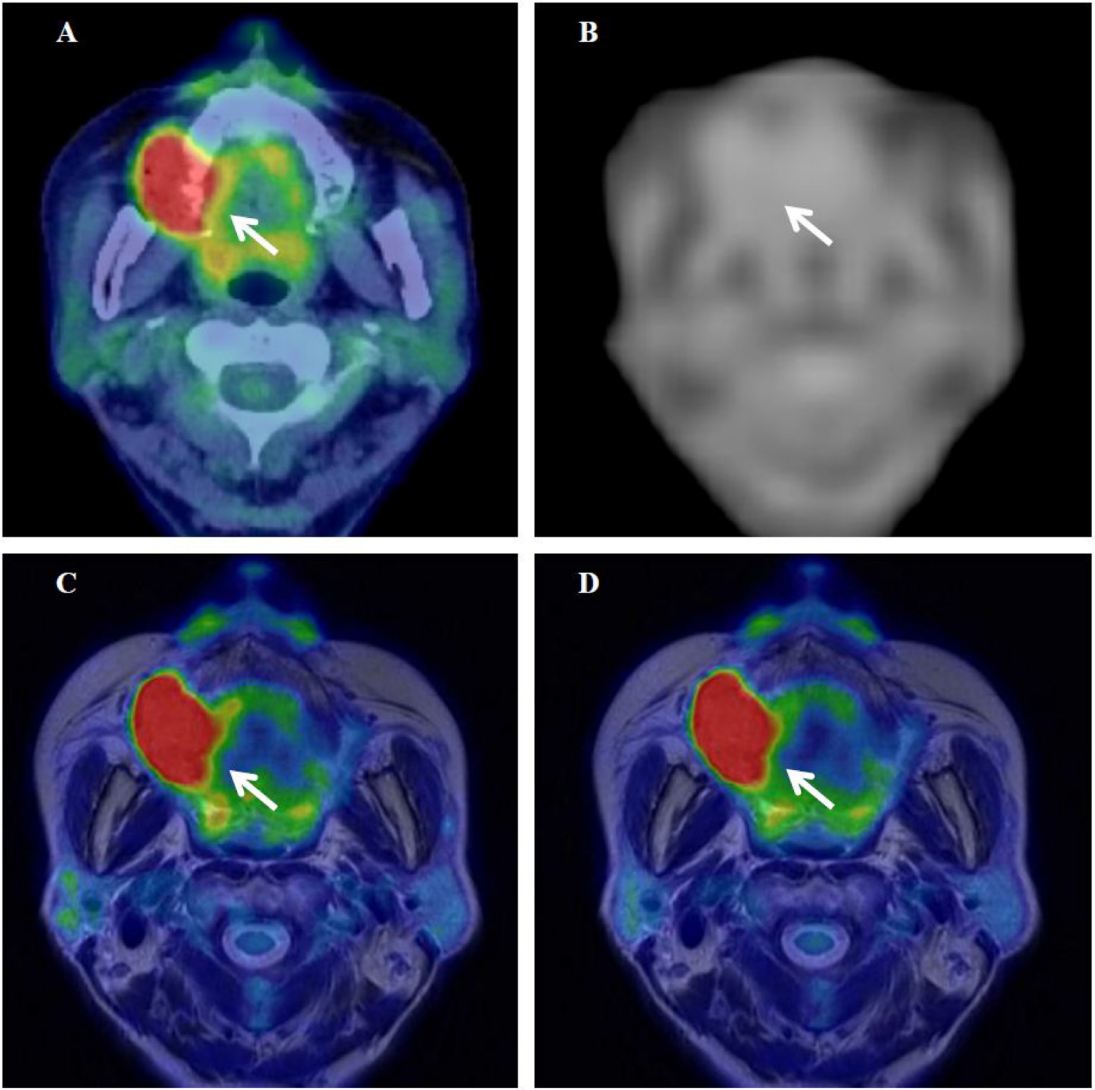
^18^F-FDG PET/CT (A), the MRAC map (B), and PET/MR_0-2min_- and PET/MR_0-10min_-T2WI fused images (C and D) of a 67-year-old woman with stage IVA upper gingival cancer. Arrows show the primary tumor.
